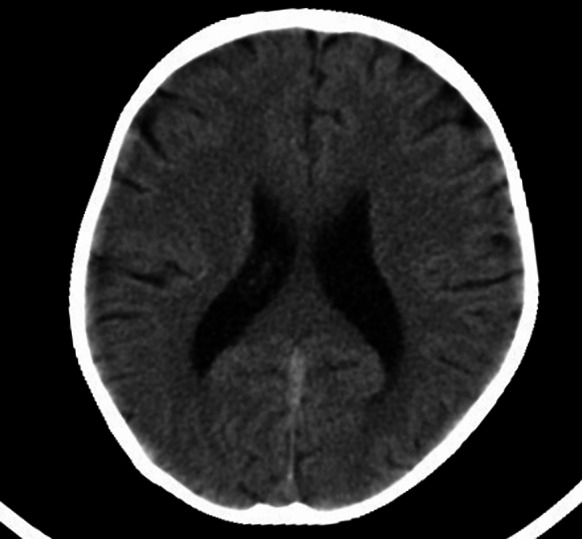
Computed tomography examination showed bilateral ventricle enlargement and sulci widening
